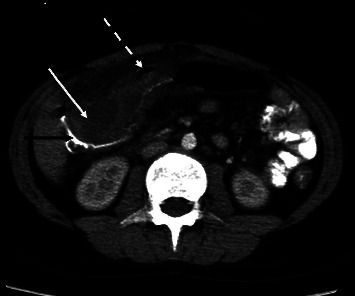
CT of abdomen and pelvis with intravenous and oral contrast showed severe wall thickening (black arrow) of the ascending colon with adjoining phlegmon (white arrow), and small amount of adjacent free air suggesting contained perforation (dotted white arrow).
